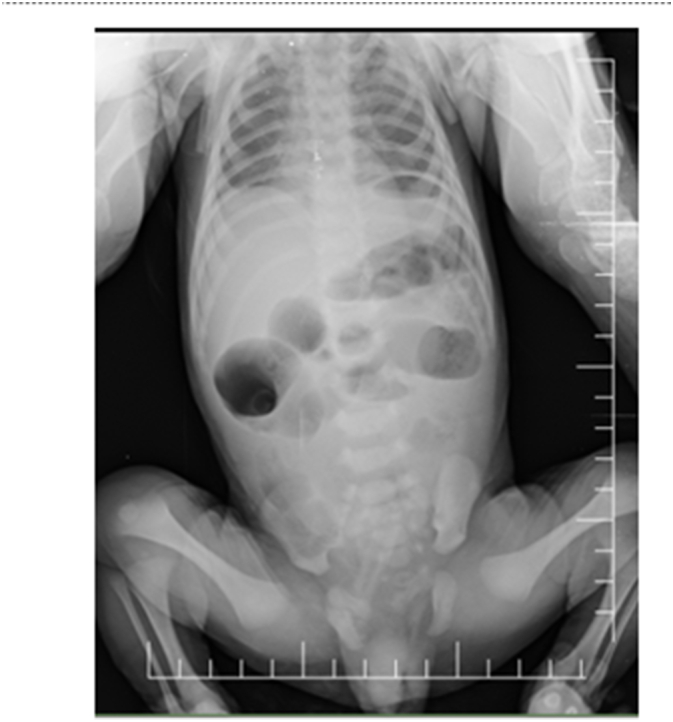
Erect abdominal X-ray with air fluid level.
